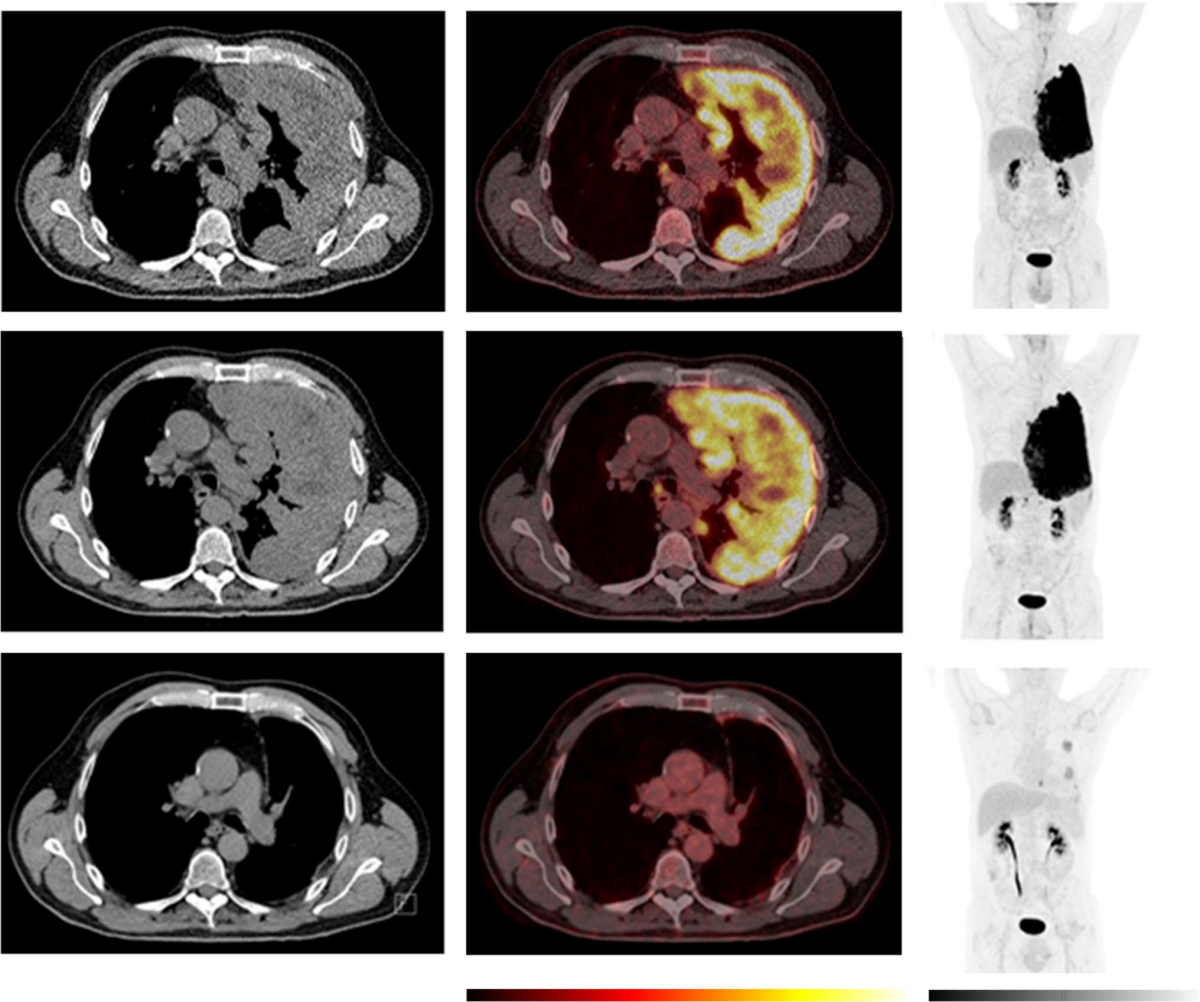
Example of a patient with a pseudoprogression. An initial increase in tumour volume and MTV and a decline in [^18^F]fluorodeoxyglucose (FDG) uptake at week-5 was followed by a long-term objective tumour response/disease control. Comparative imaging from baseline (top row), week-5 (middle row), and one-year (bottom row). To the left, axial computer tomography (CT) images, in the middle, axial positron emission tomography (PET)/CT images and to the right the maximum intensity projection (MIP). The PET images are observed within a 0-10 SUV window. The patient was classified as having progressive disease at the first CT response assessment, before developing stable disease at the week-12 and partial response at the week-18 CT scan according to the modified Response Criteria in Solid Tumours (mRECIST) and immune RECIST. At the week-5, the PET/CT showed an increase in metabolic tumour volume (MTV), while [^18^F]FDG uptake (SUV_max_ and SUV_peak_) has started to decline. At the one-year follow-up PET/CT, there is a notable change, with a visible reduction in tumour size, MTV, and [^18^F]FDG uptake. This corresponded with clinical performance status which was reduced at week-5 and improved from week 12 and onwards. The patient died due to disease progression 2 years after the start of the treatment
